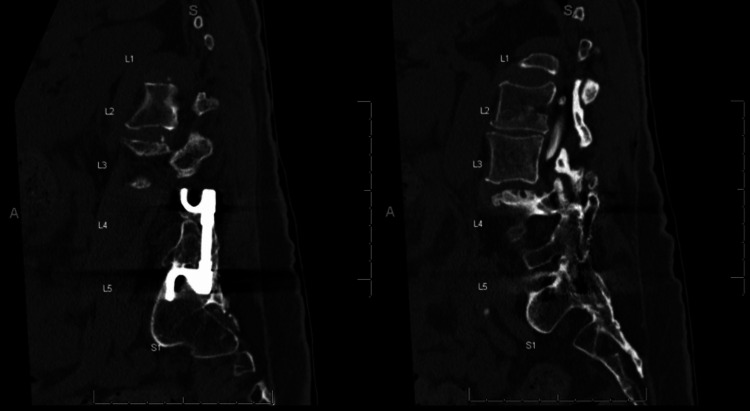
Sagittal CT scan demonstrating Harrington rod instrumentation with intact fusion mass. CT - Computed tomography
